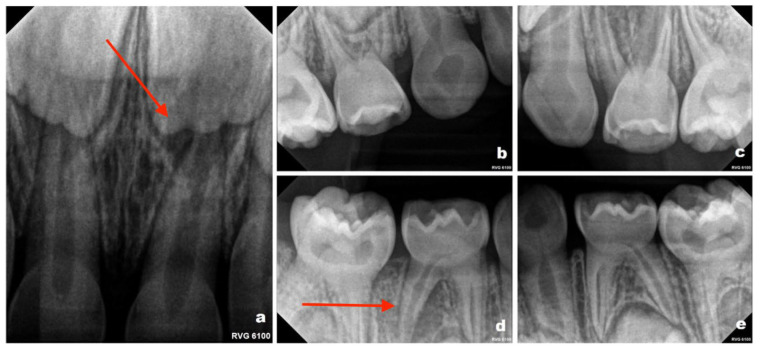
Periapical radiographs obtained in September 2022 (patient aged 3 years): (a) maxillary anterior region showing a periapical radiolucency associated with tooth 61 (Please note the area indicated by the red arrow in the figure); (b) upper right quadrant; (c) upper left quadrant; (d) lower right quadrant, demonstrating periapical changes at the distal root of tooth 84 (Please note the area indicated by the red arrow in the figure); (e) lower left quadrant.
